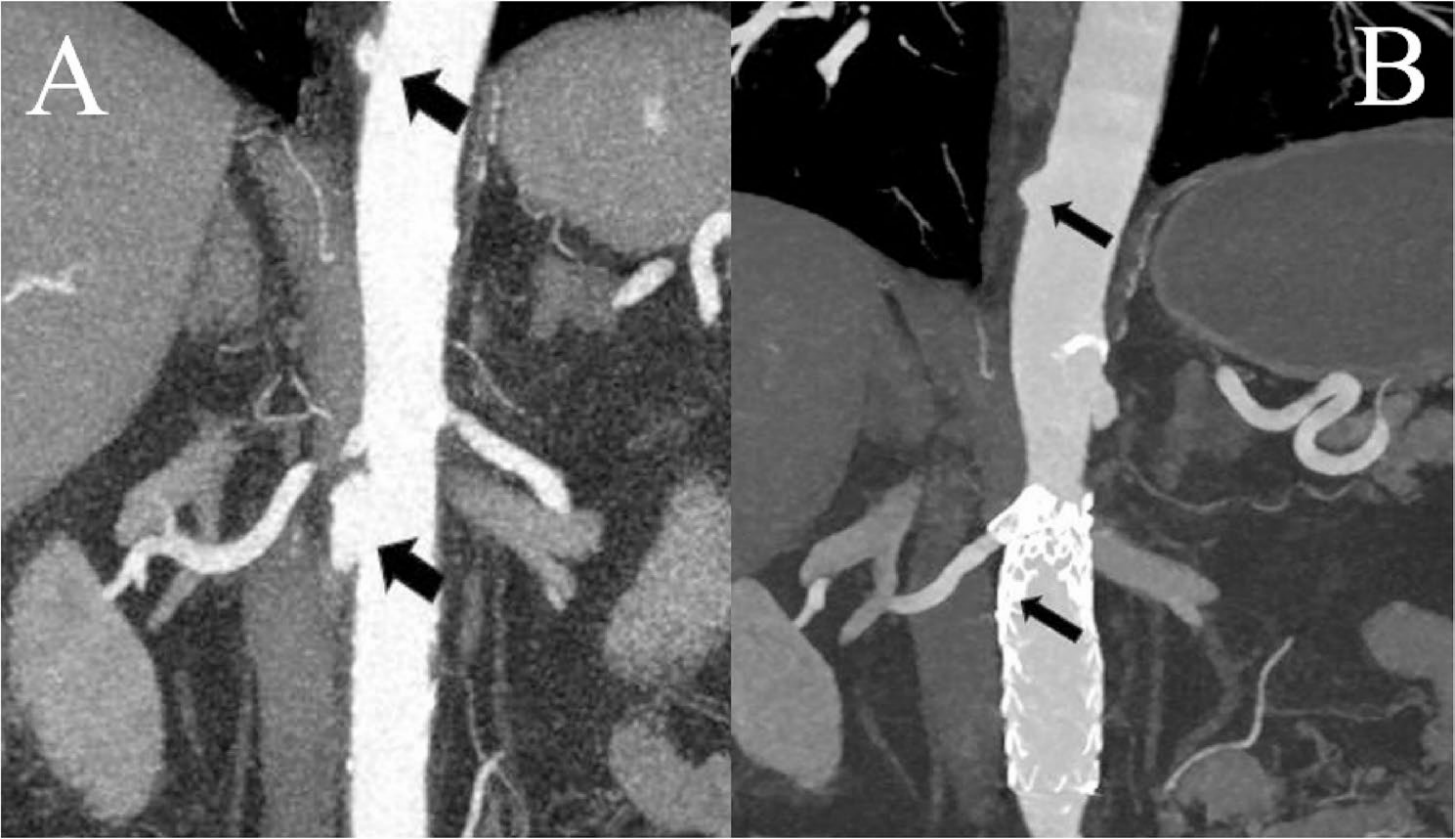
A CT abdominal aorta displaying an infrarenal, infected aneurysm and a small ulceration in the distal thoracic aorta (both marked with black arrows). B CT abdominal aorta one year postoperatively displaying a stented infrarenal aorta without a visible aneurysm and a minor ulceration in the distal thoracic aorta without inflammatory signs (both marked with black arrows)
